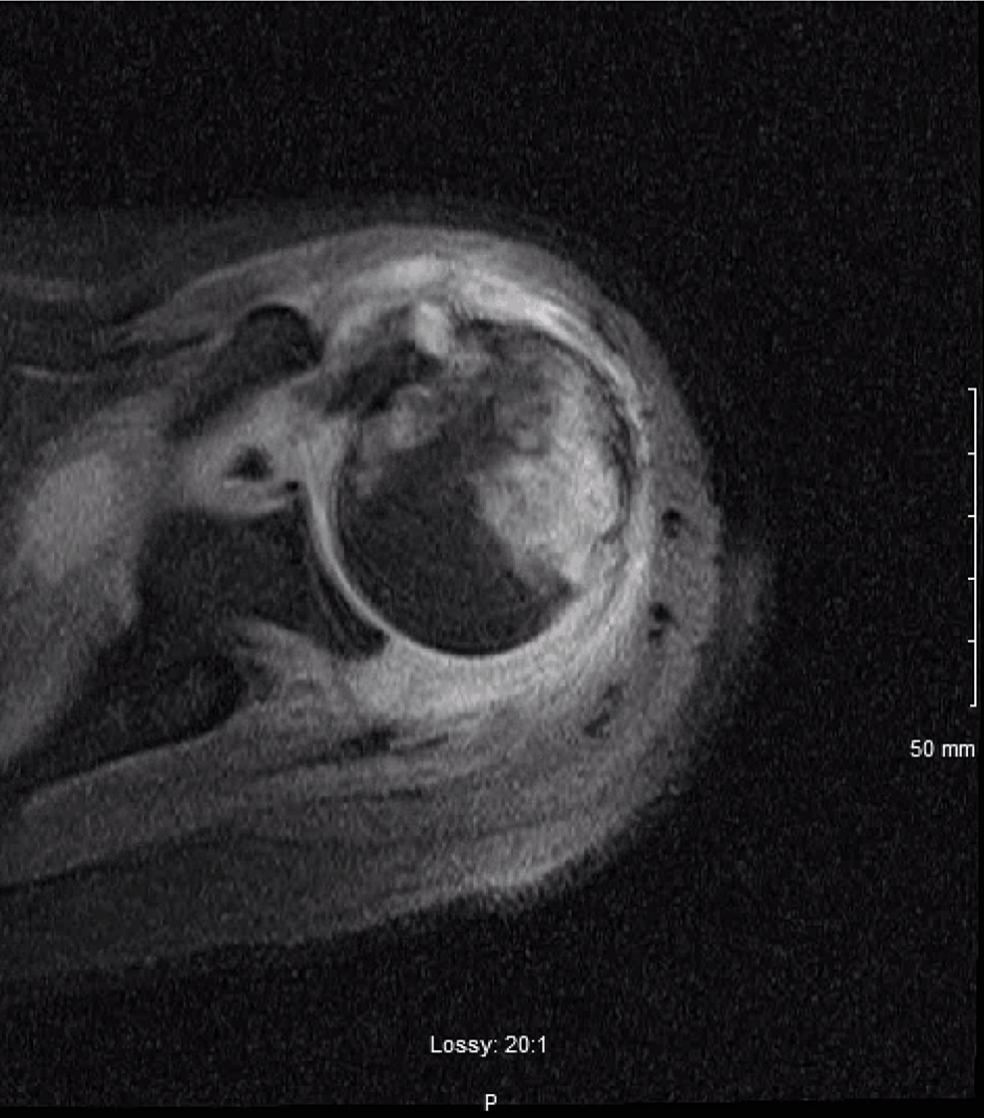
Magnetic resonance imaging (MRI) of the left shoulder Description: Non-contrast magnetic resonance imaging of the left shoulder demonstrating inflammation and edema of the joint space
